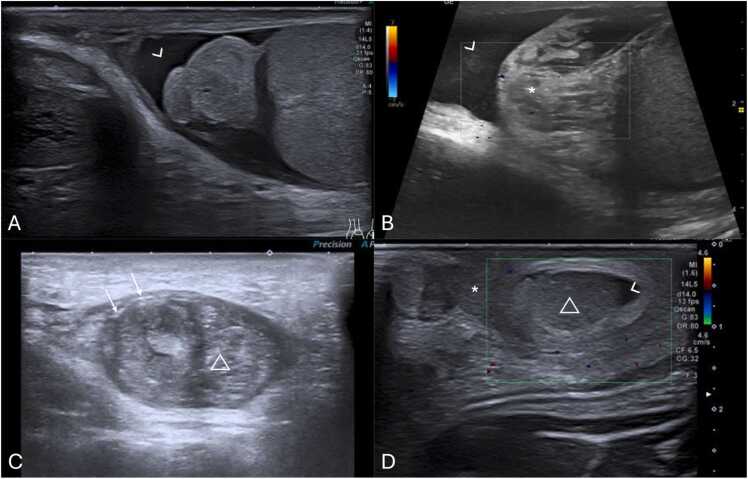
(A–D) Scrotal ultrasound of surgically confirmed testicular torsion in different patients. (A and B) Intravaginal testicular torsion in two different males, depicting a hypoechoic torsed testicle with fluid (arrowhead) surrounding both the testicle and spermatic cord (*), indicating the presence of a bell-clapper deformity. (C and D) Extravaginal testicular torsion in different patients, depicting atrophied testis (△) with an heterogenous parenchymal structure with associated linear calcifications of the tunica albuginea (arrows in C). Notice the presence of fluid extending along part of the subtunica of the torsed testicle (arrowhead in image D), without the presence of fluid surrounding the spermatic cord (* in D), suggestive of extravaginal torsion.
